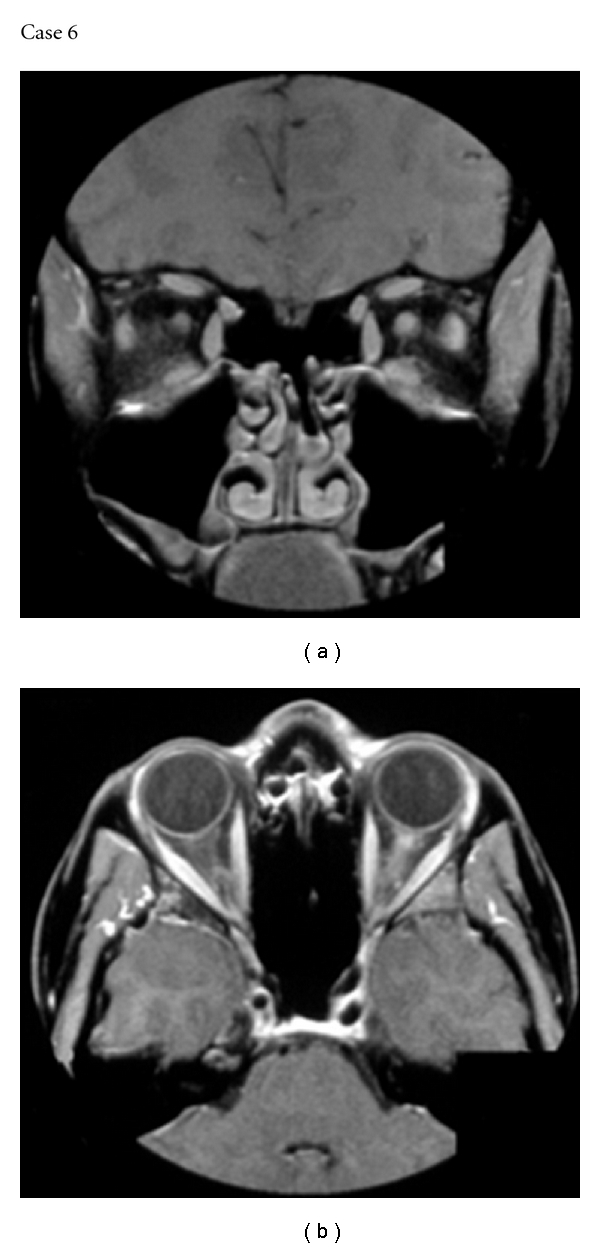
MR images of Case 6 showing gadolinium enhancement of the left optic nerve 24 hours after the onset of left retroorbital pain.
